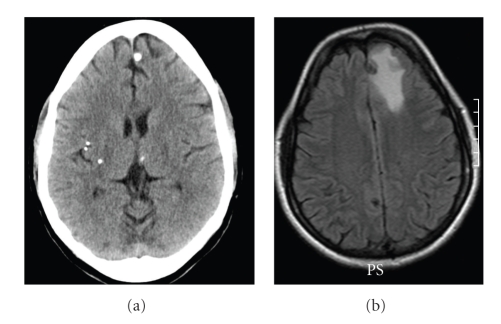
(a) Baseline CT scan demonstrating a dense calcification in the left frontal lobe as well as other calcifications. (b) MRI images that used fluid attenuated recovery after the patient had a seizure revealing perilesional edema.
